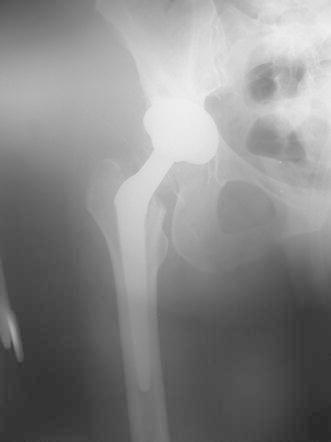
Radiographic aspect at 1 year follow-up from total hip arthroplasty showing correct placement and good integration of the implant
